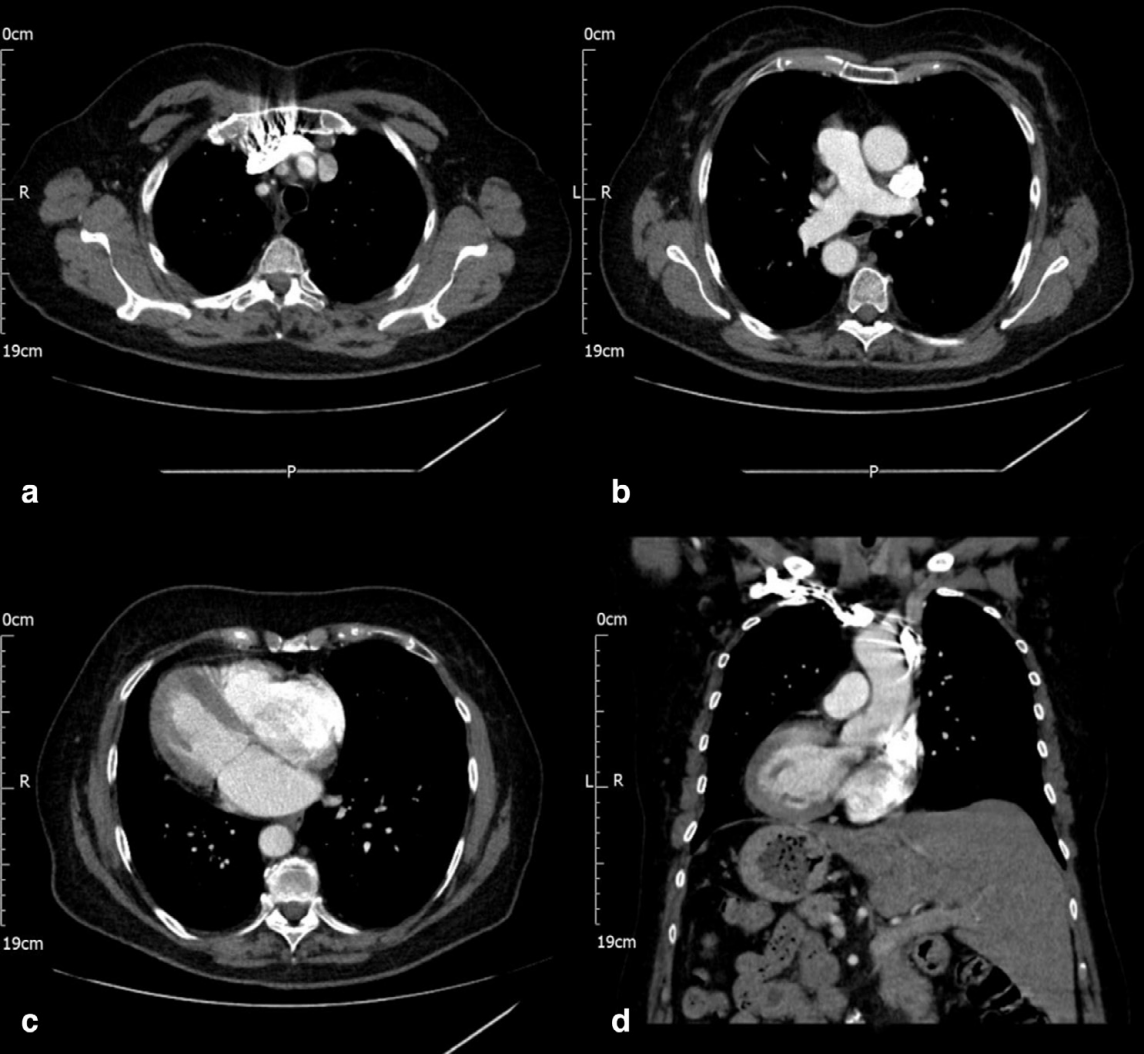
CT scan of the chest shows (a) SVC and brachiocephalic trunk to the left, (b) pulmonary trunk to the right, ascending aorta to the left and descending aorta to the right, (c, d) heart in the right hemithorax and liver on the left side of the abdomen. SVC, superior vena cava.
